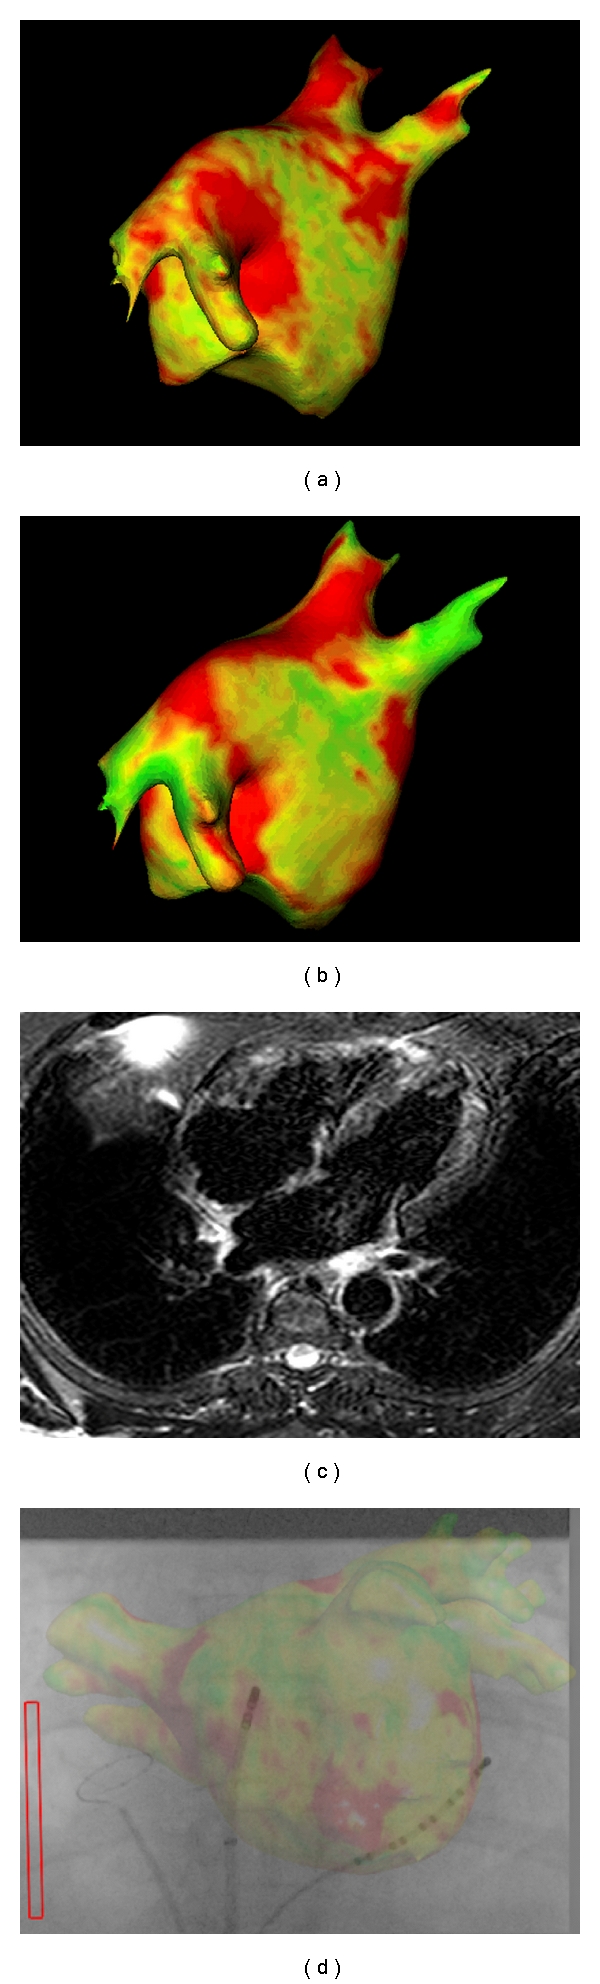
Left atrial magnetic resonance imaging. Postprocedural imaging of the left atrium with delayed enhancement ((a, b)) and T2 weighted (c) to assess left atrial scar and oedema, respectively. The raw image is processed to obtain a 3D representation of the anatomy, with colour-coded representation, which is thought to represent scar formation and oedema. The images may help guide ablation (d). Images courtesy of Ben Knowles and Kawal Rhode, Kings College London and St. Thomas' Hospital.
